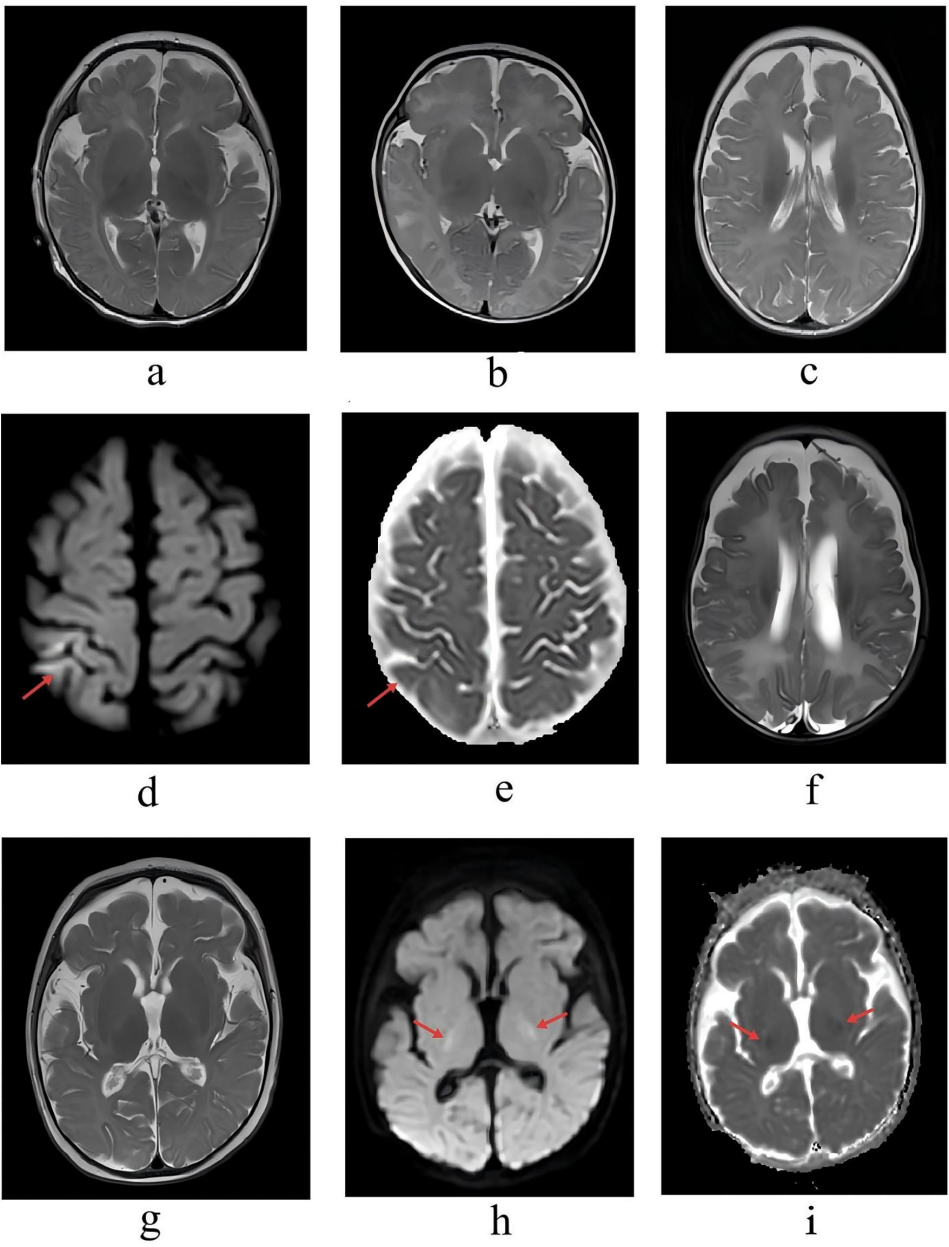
Cranial MRI (including DWI) findings during ICU admission. (a,b) Slight widening of frontal and temporal extracerebral spaces in Cases 1 and 2. (c) Mild bilateral ventricular enlargement, slight frontal extracerebral space widening, and slight corpus callosum thinning in Case 3. (d,e) Slight bilateral ventricular enlargement, with high DWI signal and corresponding ADC mild hypointensity in the right parietal lobe in Case 4. (f) Widened extracerebral spaces in bilateral frontal and temporal regions and the cerebral longitudinal fissure in Case 5. (g–i) Widened cerebral sulci and fissures, slightly high DWI signals in bilateral posterior limbs of internal capsules, and notably low ADC signal in Case 6.
